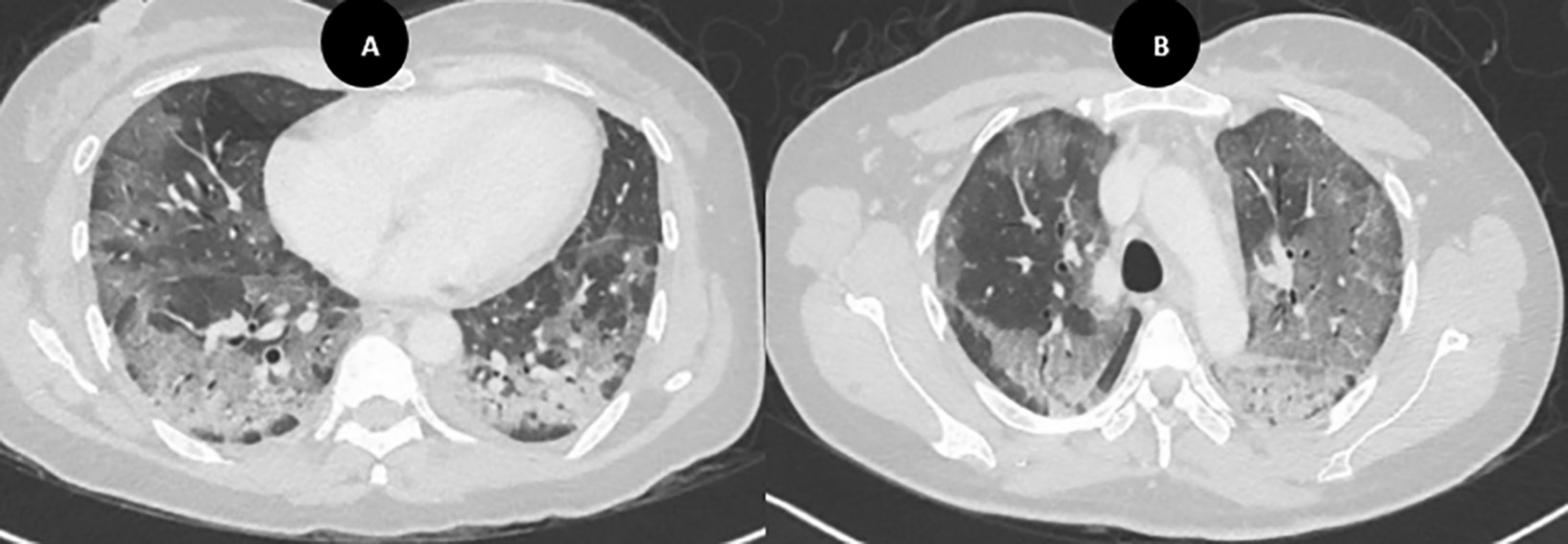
(A, B) Initial HRCT performed on day 3 of admission shows bilateral, diffuse, patchy ground‐glass opacities with subtle interlobular septal thickening.
